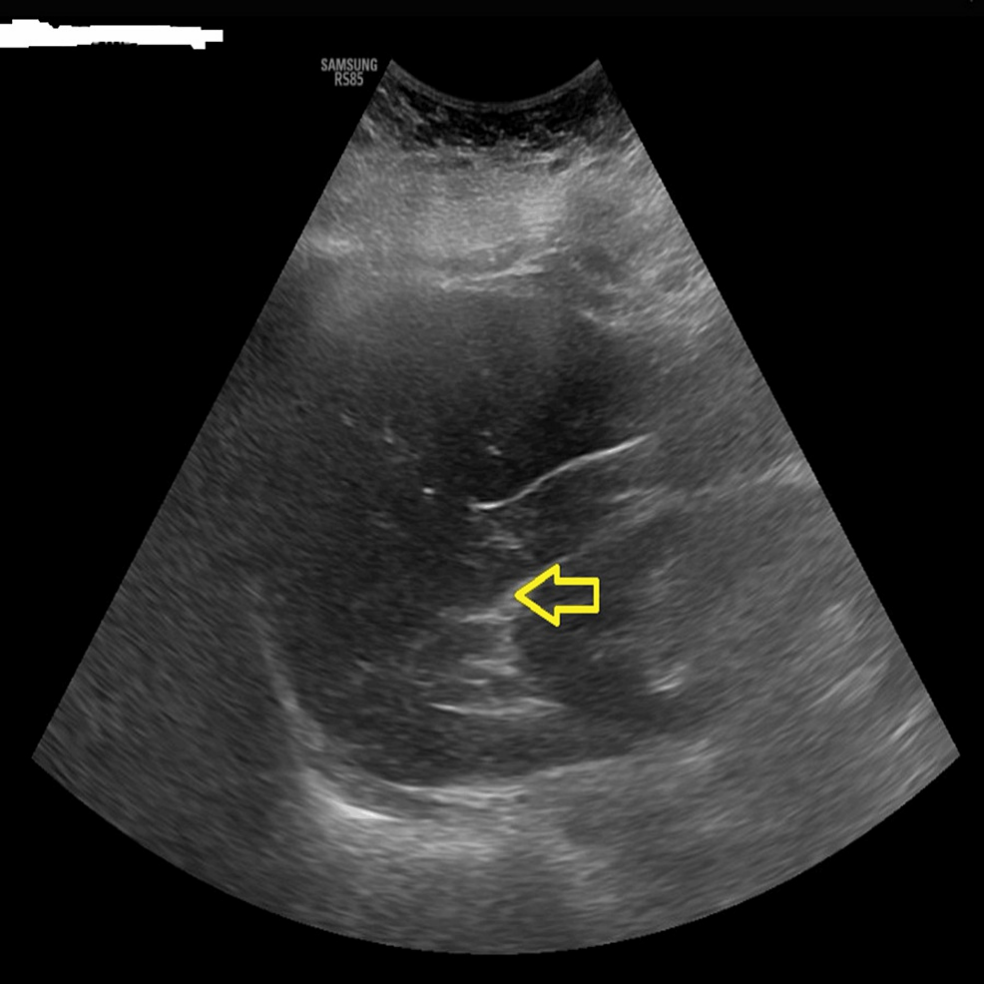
Splenic ultrasound showing negative for structural splenic abnormalities
